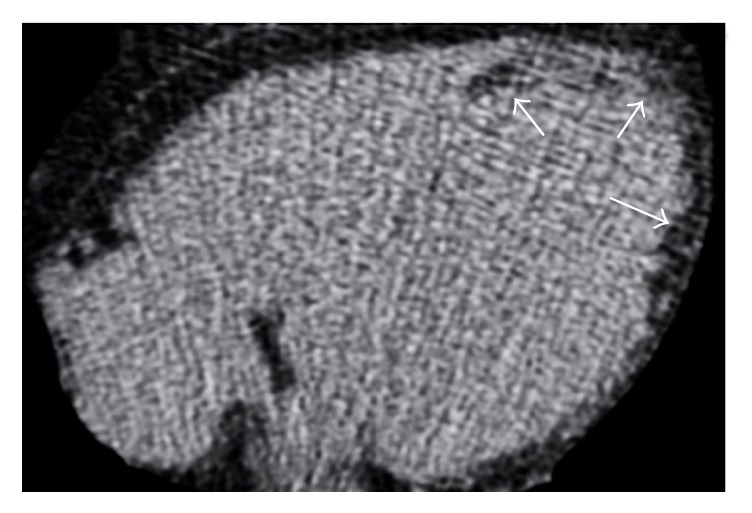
Cardiac CT axial image without contrast media administration in a 72-year-old male after an extensive chronic myocardial infarction shows the presence of a curvilinear hypodense stripe (arrows) with negative attenuation values (−20 Hounsfield units), located within the subendocardial layer of the left ventricular apex extending also to the left ventricular lateral wall, findings related to a postischemic lipomatous metaplasia.
